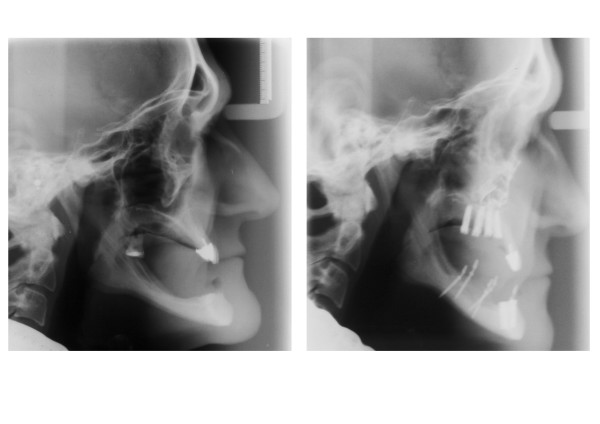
Preoperative (left) and postoperative (right) radiographs: improvement of the intermaxillary relationship after orthognathic surgery.
